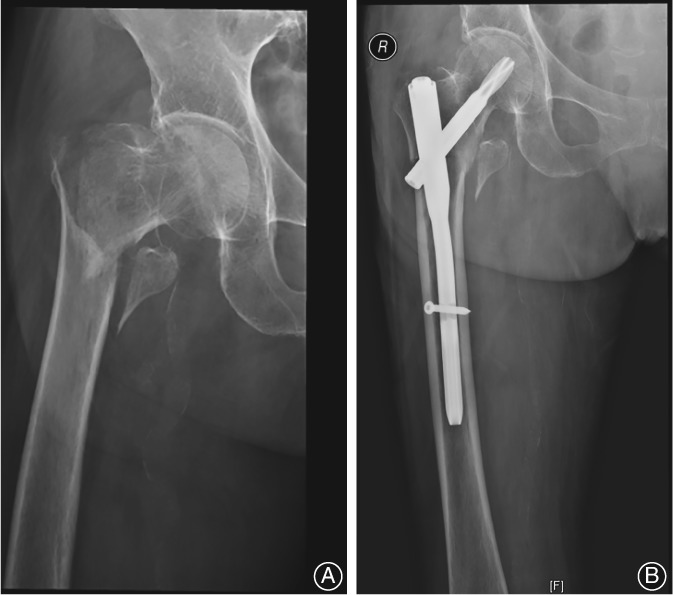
(A) Anteroposterior radiograph showed an unstable intertrochanteric fracture. (B) Radiograph made 1 week after fixation with proximal femoral nail anti‐rotation.
